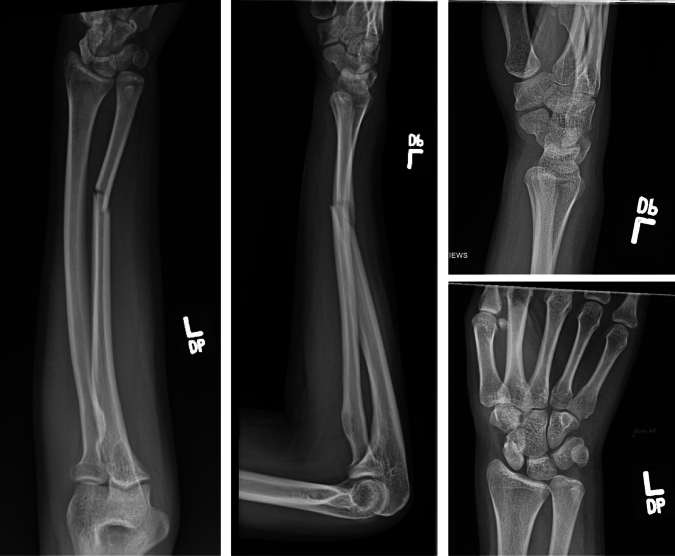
Injury radiographs demonstrating a displaced and translated midshaft ulna fracture, concern for DRUJ injury, and positive ulnar variance
